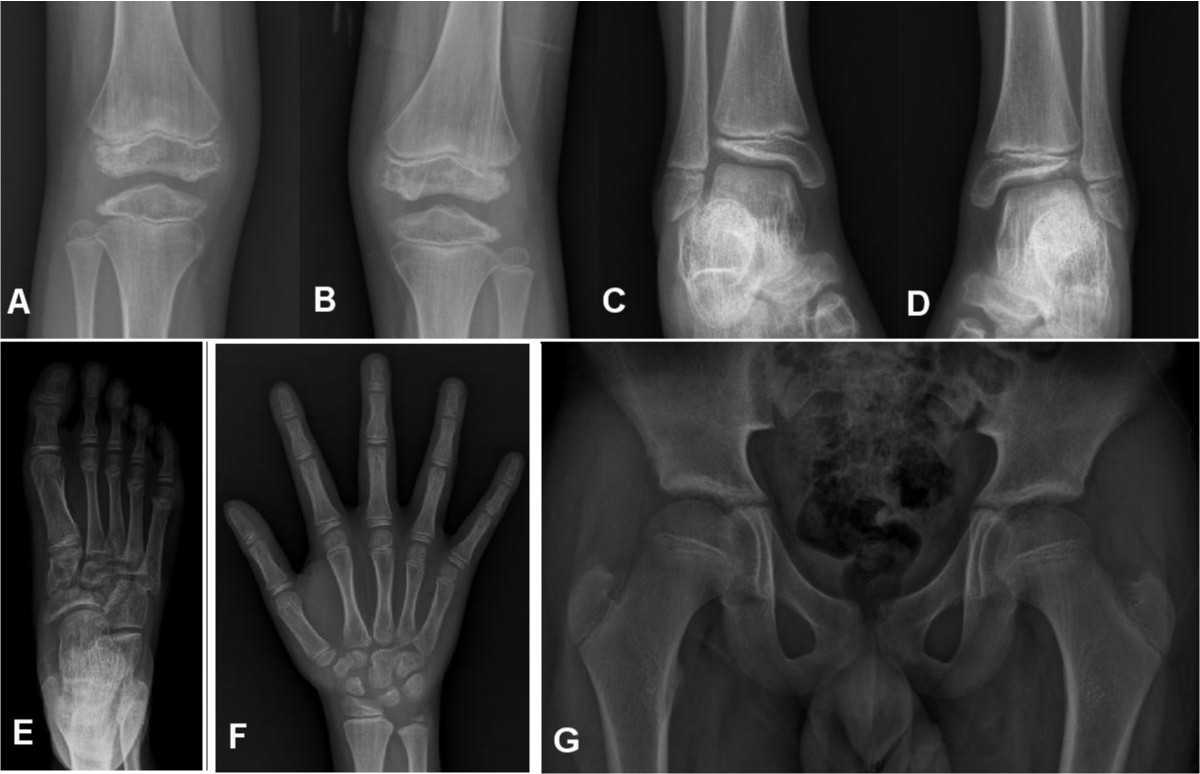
Radiographs of proband at the age of 12 years. Anteroposterior view of knee joint showed shortening, fragmentation, and joint surface irregularity of distal femoral and proximal tibial epiphysis (A, B). Anteroposterior view of distal tibial epiphysis showed a lateral wedging and fragmentation (C, D). The tarsal navicular and cuneiform bone showed irregular ossification (E). The epiphysis of distal radius was wedge shaped and the epiphyses of the distal ulnae were relatively small and the carpal bone shows dysplasia and flattening (F). The epiphyses of proximal femur were spared (G).
